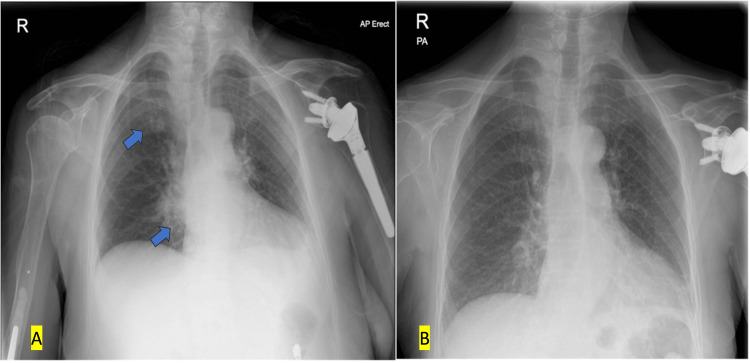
A: chest x-ray on current admission showing a nodule in the right upper zone with a bulky right hilum (blue arrows). B: old chest x-ray for comparison taken approximately one year ago. Panel A: New right-sided lung opacities highlighted by blue arrows. Panel B: Old chest x-ray showing showing absence of any abnormal lung findings.
